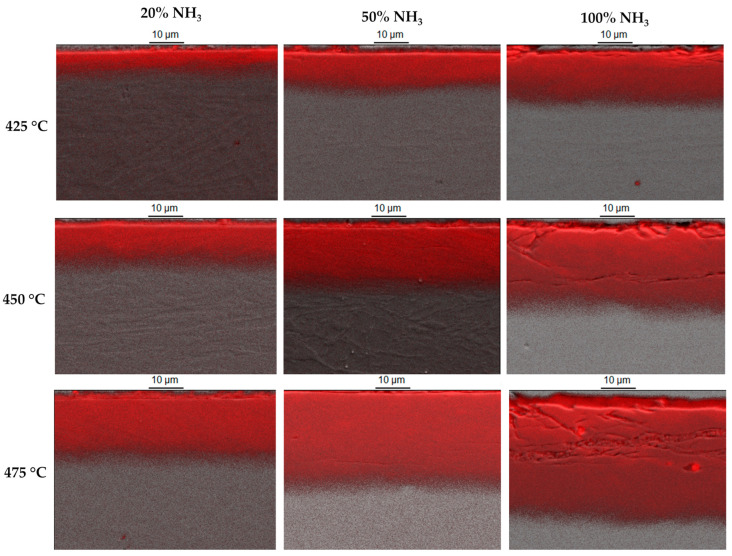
Qualitative distribution of nitrogen (red colour) on cross sections of nitrided layers on Nanoflex steel under condition C with various treatment parameters (treatment time: 4 h). Secondary electron image and WDS X-ray microanalysis.
